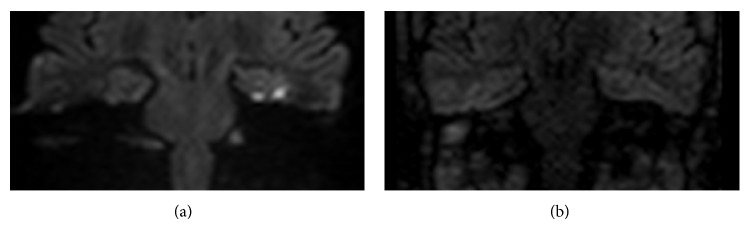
Images of right middle ear cavity in patient with clinical suspicion of recurrent cholesteatoma: example of SSh SE-EPI DWI false negative (a) compared to MSh TSE DWI true positive (b).
